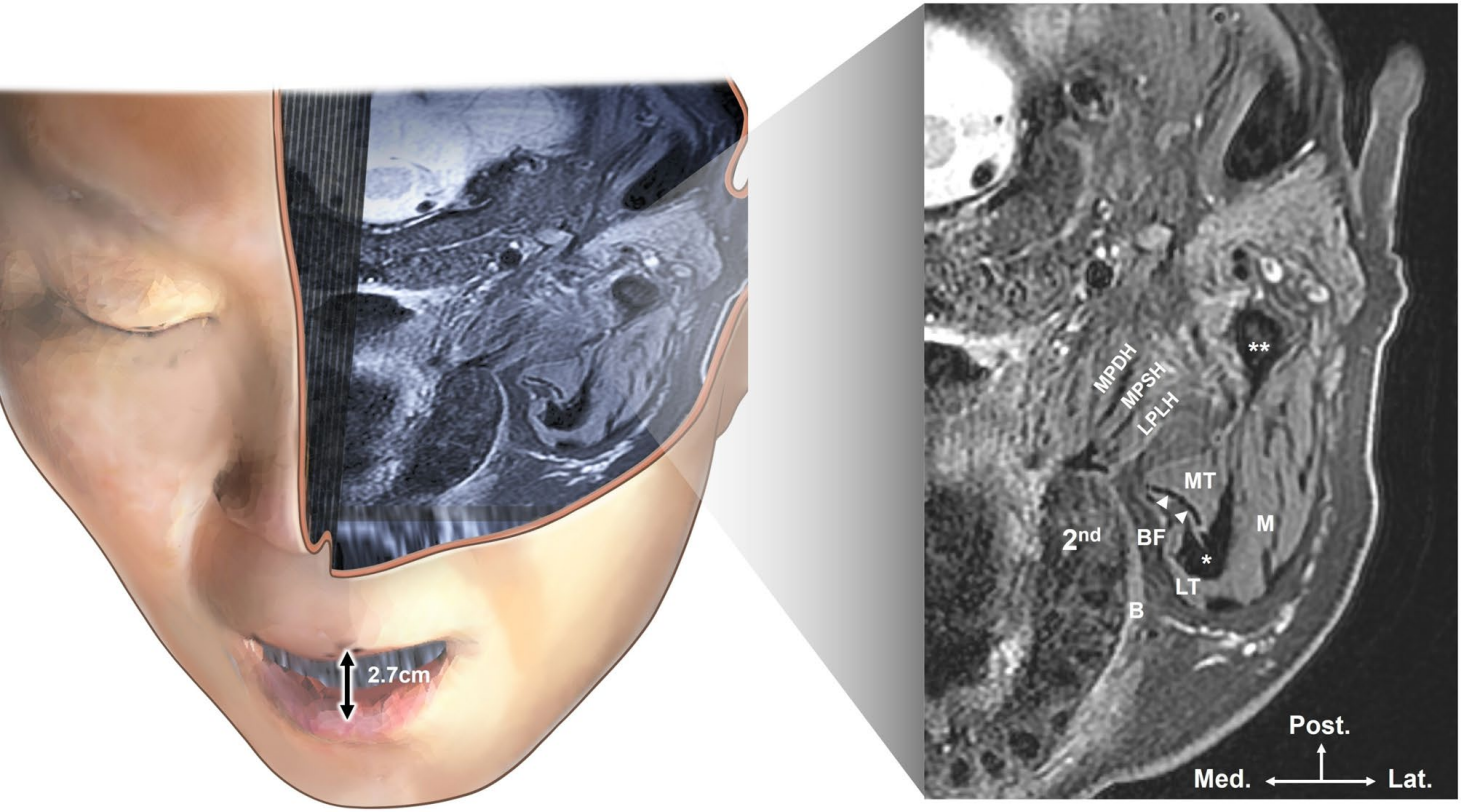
Superimposed model of the three-dimensional scanned face and magnetic resonance imaging (MRI), and MRI structures observed at the reference point level. second, maxillary second molars; MPDH, deep head of medial pterygoid muscle; MPSH, superficial head of medial pterygoid muscle; LPLH, lower head of lateral pterygoid muscle; B, buccinator muscle; BF, buccal fat pad; MT, medial portion of temporalis muscle; LT, lateral portion of temporalis muscle; M, masseter muscle; Asterisk, coronoid process; Two asterisk, condylar process; arrowhead, temporalis tendon; Med, medial; Post, posterior; Lat, lateral.
